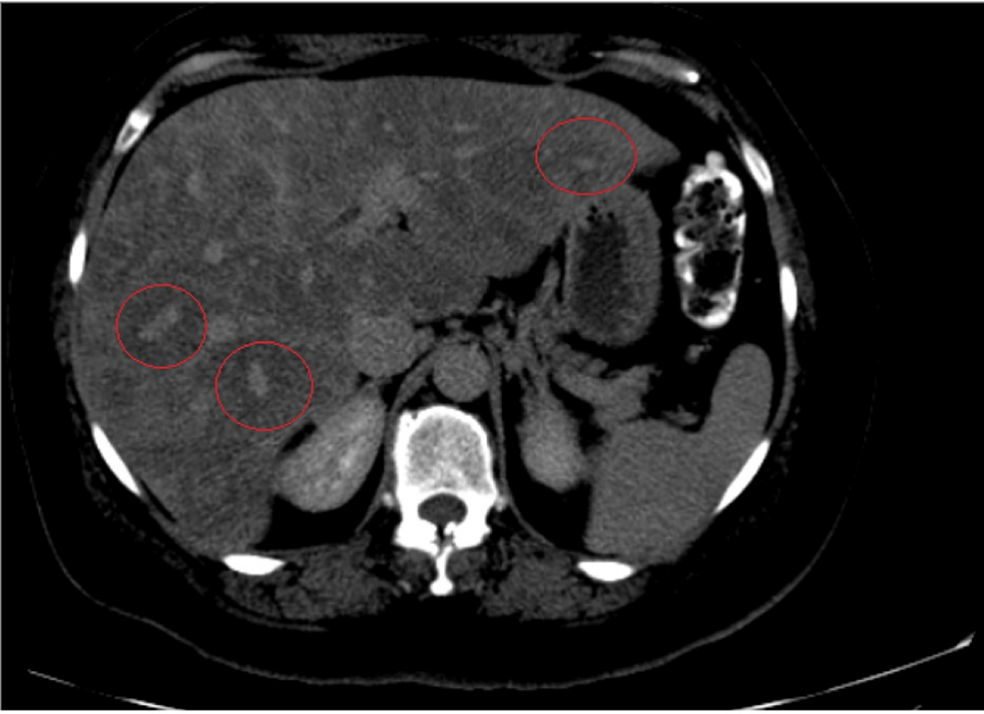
Hepatic hypodensities. CT of the abdomen and pelvis with contrast showing decreased attenuation with diffuse hypodensities (red circles), suggesting spared areas but no clear nodules in the liver.
